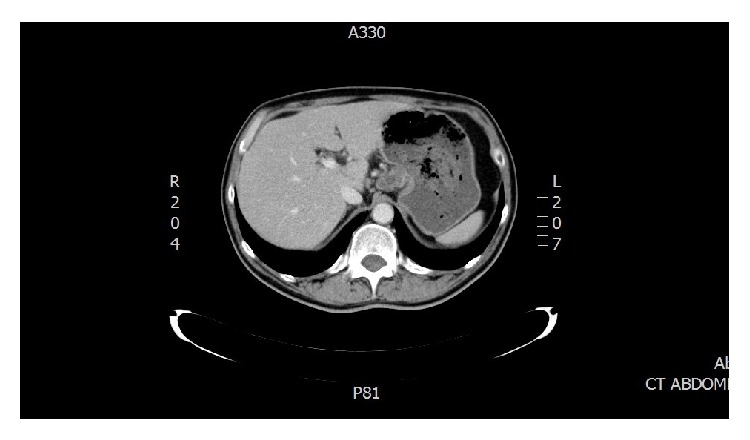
Computed tomography scan (CT) showed a structure of 2.0 × 3.5 cm in diameter on a lesser gastric curvature that could stand for large penetrating ulcer.
